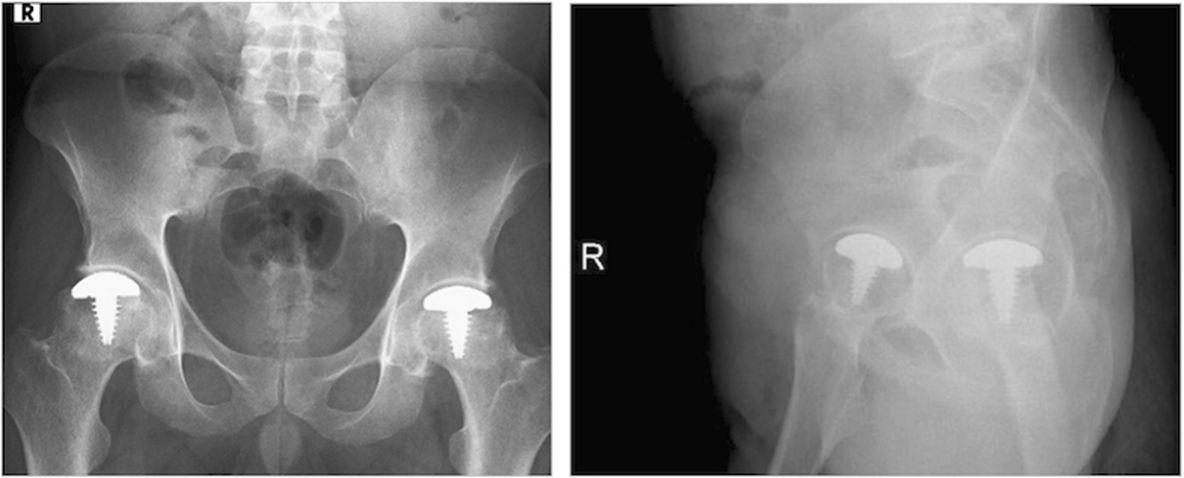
Postoperative X-rays. X-rays at postoperative second years of a 48-year-old male patient. These radiographs show the preservation of the joint spaces without loosening of the implants bilaterally. The screws were removed because of irritation in this patient.
